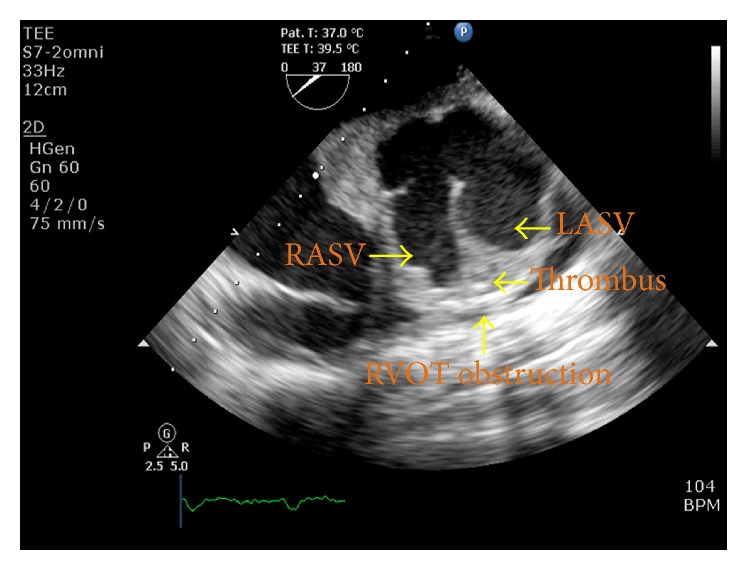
Transesophageal echocardiography^*∗*^ revealed left aneurysm of sinus of Valsalva (LASV) and right aneurysm of sinus of Valsalva (RASV) with thrombus within it, causing RVOT obstruction. ^*∗*^Aortic valve short-axis view at midesophageal level.
